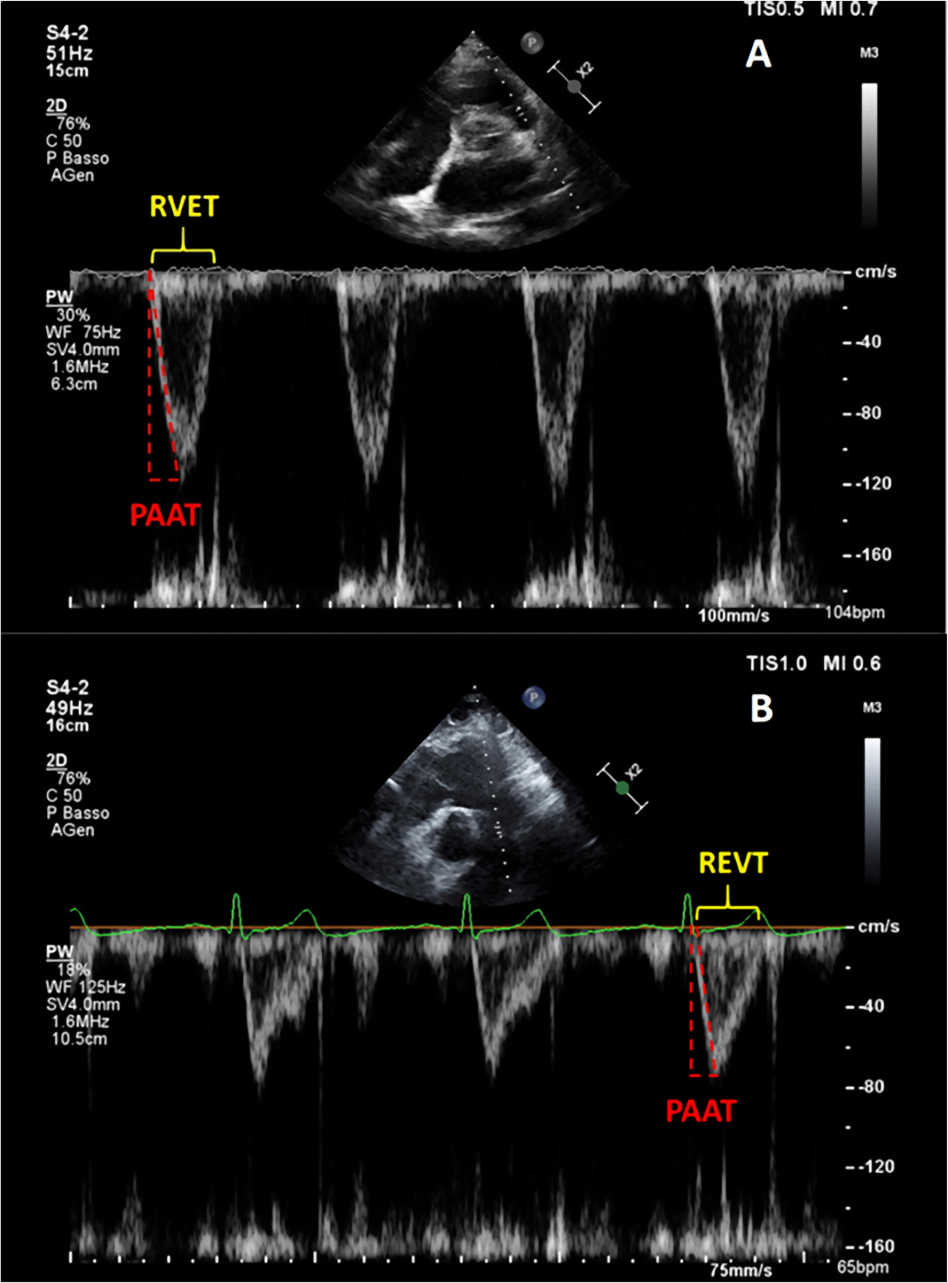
Pulmonary artery acceleration time (PAAT, dashed red line) and right ventricular ejection time (RVET, yellow brace) measurement in parasternal short-axis view (A) and subcostal view (B). PAAT is calculated as the time interval between the onset of systolic pulmonary artery flow and its peak flow velocity; RVET is measured from the onset to the end of pulmonary artery Doppler spectrum. For further adjustment, sPAP was derived on the basis of the linear correlation linking PAAT to TRV_max_ as follows: log_10_(sPAP) = −0.004 (PAAT) + 2.1 [8]. Mean PAP (mPAP) can be estimated using the following formulae proposed by Dabestani et al. [28]: if PAAT ≥ 120 ms, mPAP = 79 – (0.45 * PAAT); if PAAT < 90 ms, mPAP = 90 – (0.62 * PAAT)
